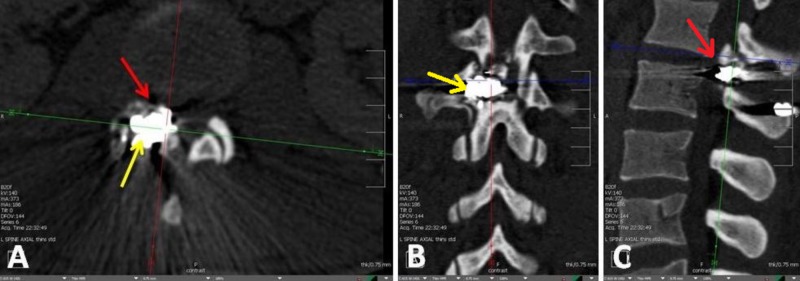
A) Axial CT image of the lumbar spine with bullet fragment lodged on the right side between the L1 and L2 laminae, causing a fracture through the right L2 pedicle with mass effect, compression of the distal roots and conus. In this image, one can view both the large bullet fragment (yellow arrow) and the neuroforaminal and recess compromise (red arrow). B) Coronal plane view. C) Sagittal plane view.
